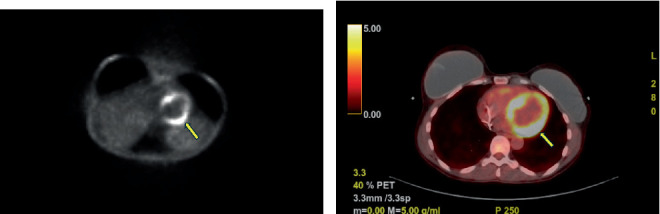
F-18 fluorodeoxyglucose (FDG) PET CT scan. (a) Two-dimensional nonattenuation correction (NAC) image and (b) color scale image demonstrate diffuse uptake of FDG in the left ventricular myocardium with increased asymmetric uptake in the posterior left ventricle and left ventricular septum as noted by the yellow arrow.
